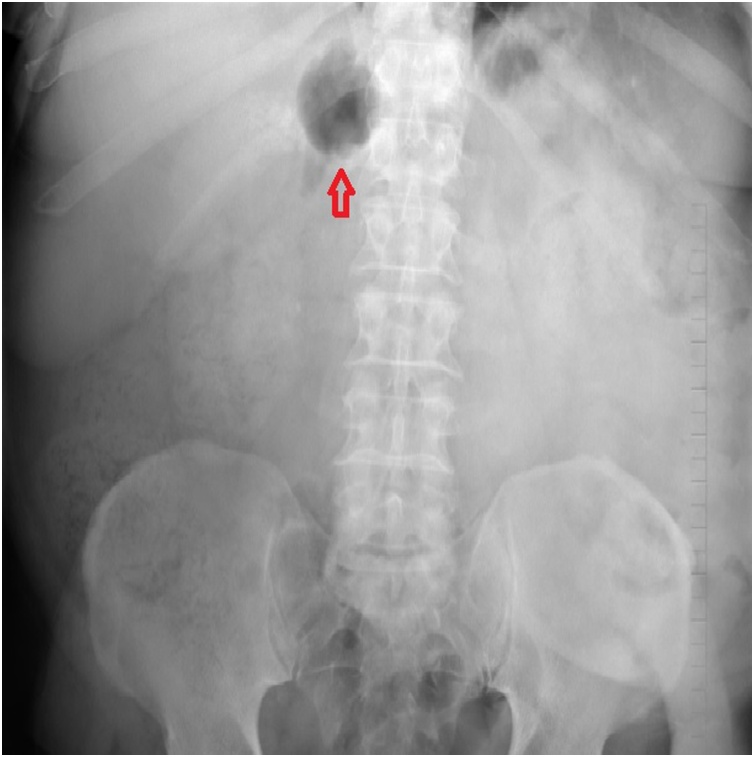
Abdominal x-ray showing a focally dilated bowel loop in the right upper quadrant (red arrow); the so called ‘sentinel loop’ sign.
